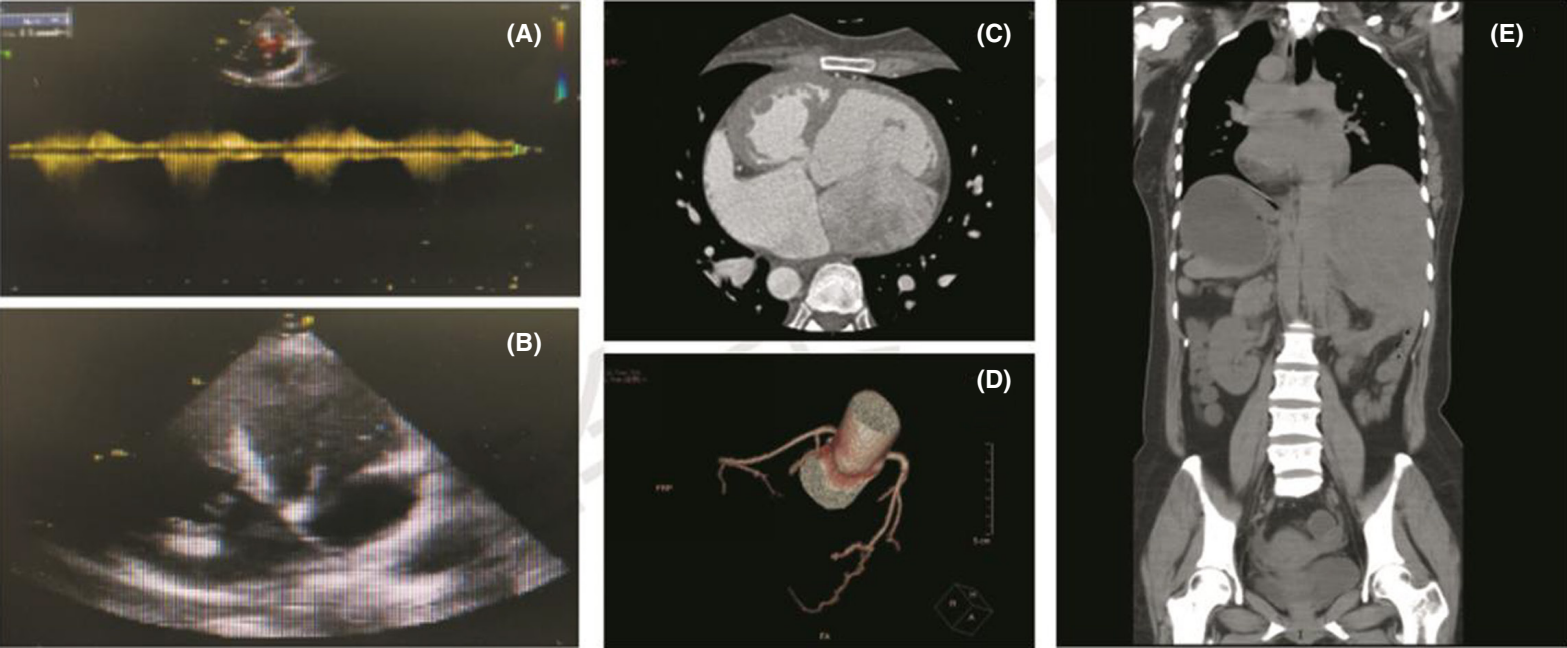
(A, B) Ultrasonic cardiogram. (C, D) Computed tomography cardiac angiography. (E) Chest, abdomen, and cavity of pelvis CT and three‐dimension image suggest total situs inversus, in which the stomach is located on the right side and the liver on the left
